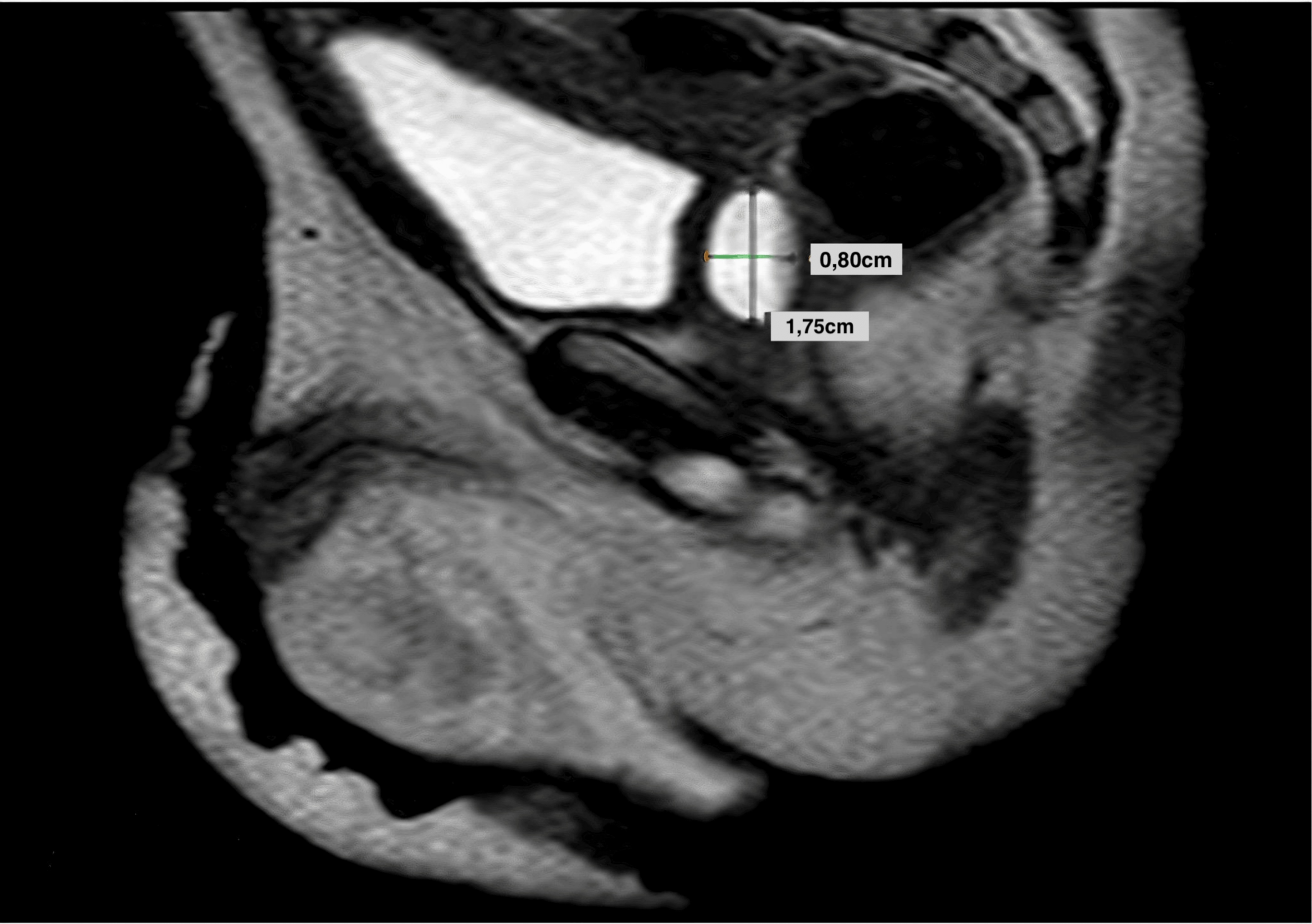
Magnetic resonance imaging prior to surgery shows the anatomical definition of the prostatic utricle
